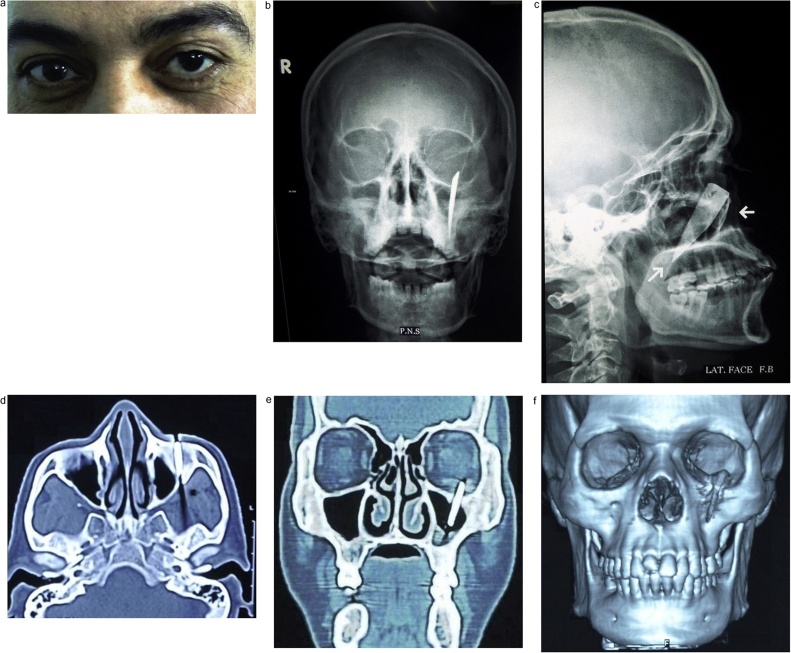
Showing the preoperative presentation of the patient with the foreign body in situ. (a) frontal view of the patient with unequal eye level. (b, c) Paranasal sinuses and lateral skull views respectively. (d, e, f) Axial, coronal and 3D computed tomography cuts respectively.
